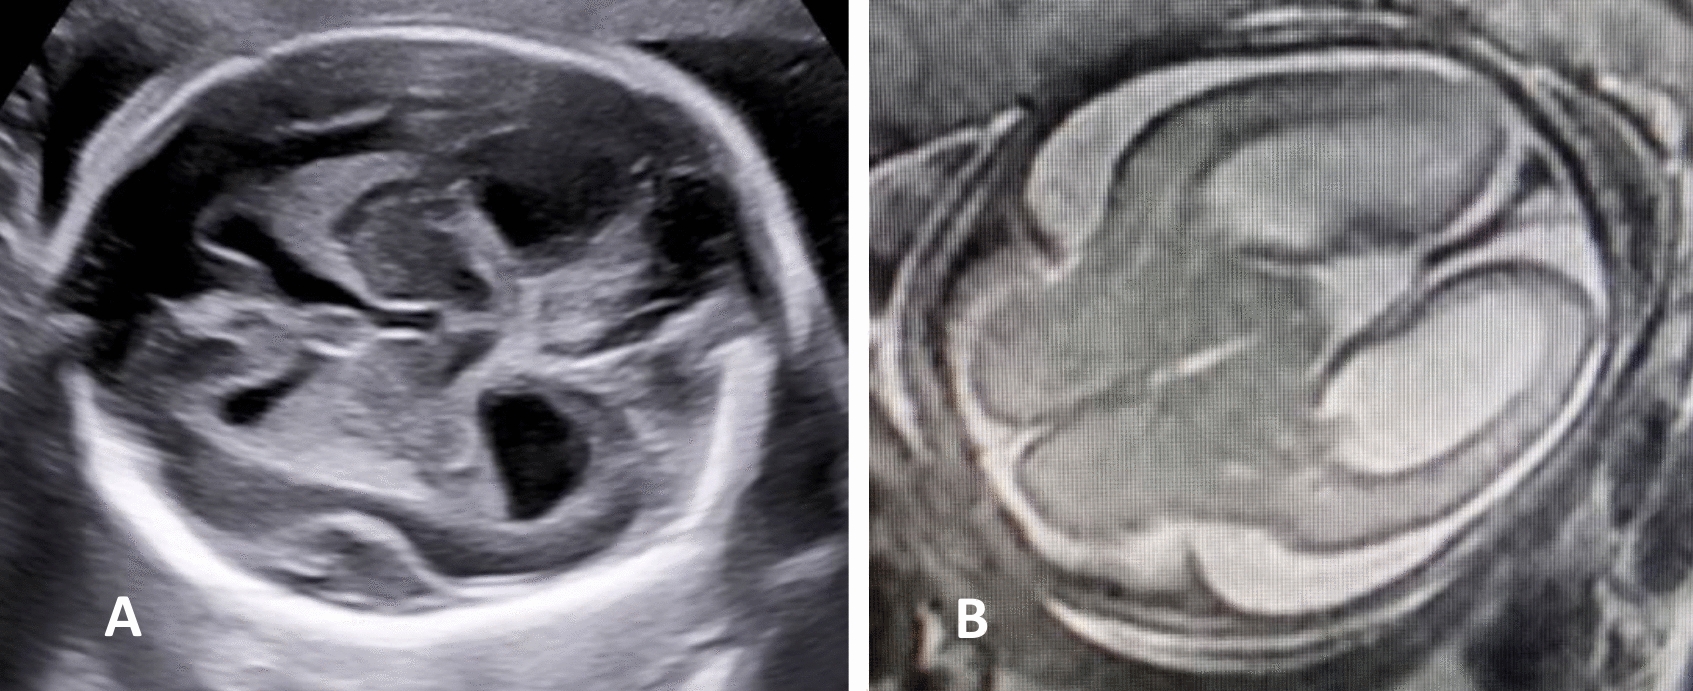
Patient 38, the fetus of a 32-year-old woman, had bilateral delayed SF and hydrocephalus. A Axial plane at 24.9 weeks by ultrasound showing delayed and trapezoid-shaped SF and bilateral hydrocephalus. B Axial plane at 25 weeks by MRI showing bilateral delayed SF and bilateral hydrocephalus
